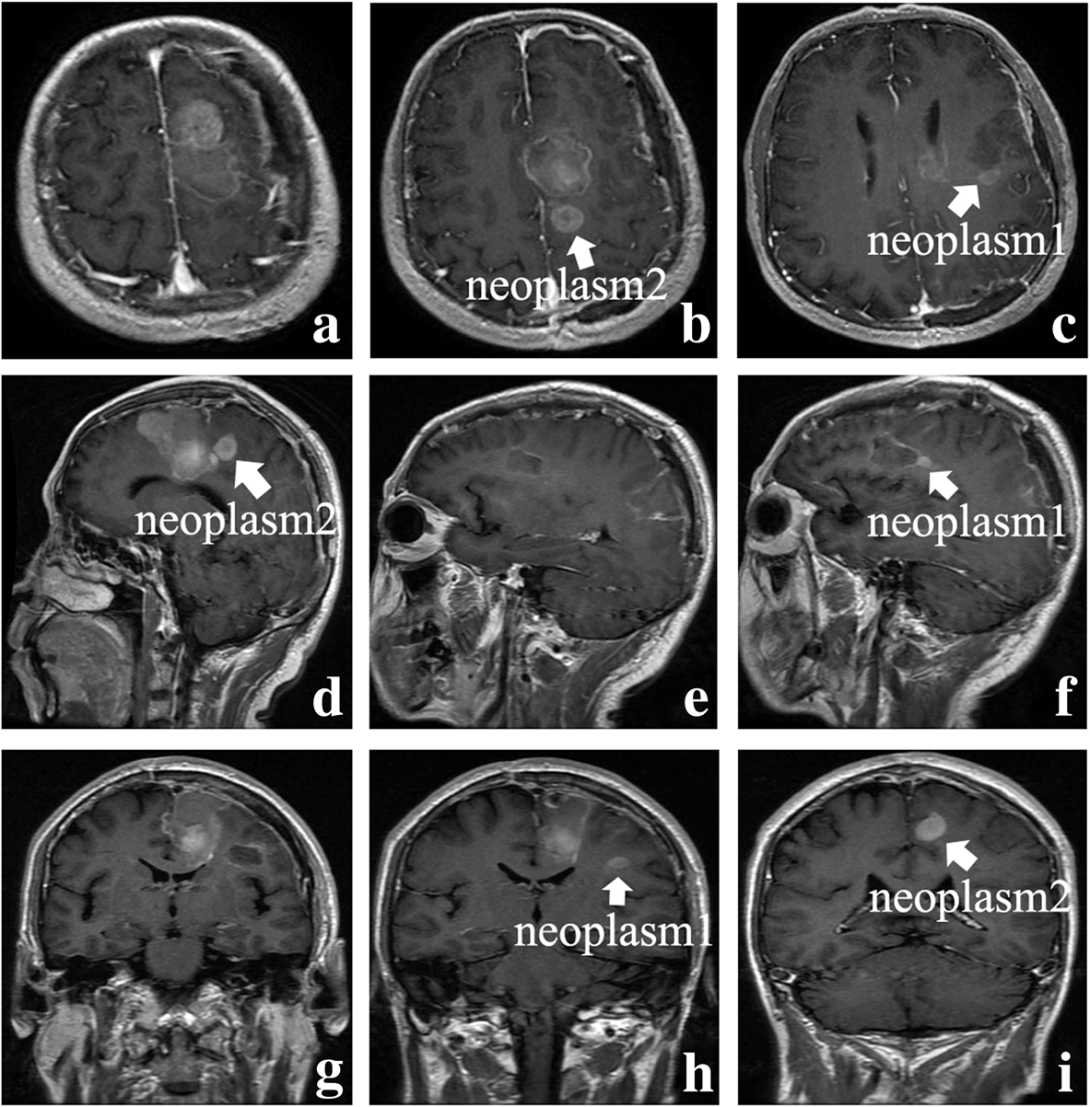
The axial, sagittal, and coronal contrast-enhanced MRI images after 5 weeks of surgery (a–i). Two new lesions occurred adjacent to the primary lesion area (arrows represent the neoplasms)
